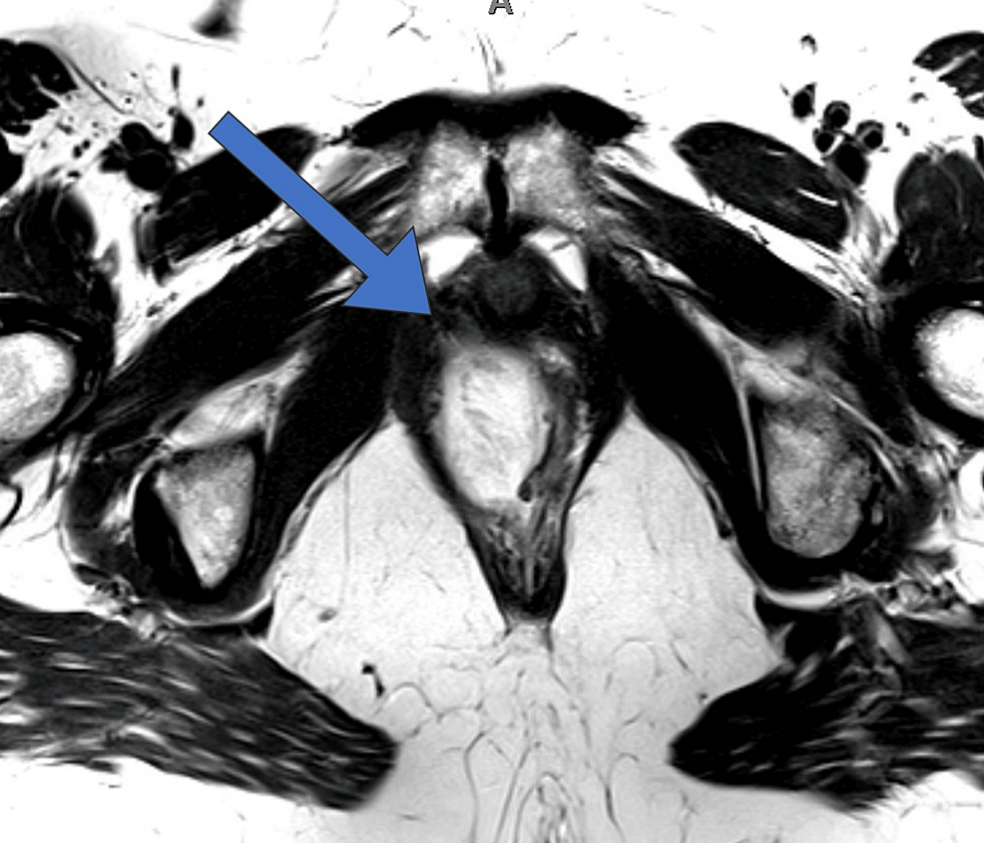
MRI demonstrating a pelvic mass with an arrow demonstrating communication to the lower rectal lumen at the 10:00 - 12:00 position
